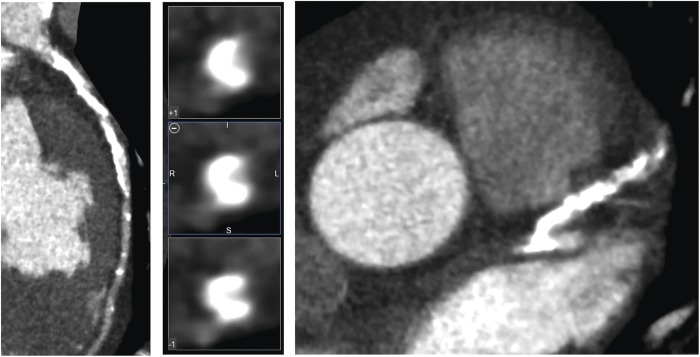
CT coronary angiography images of a heavily calcified left anterior descending artery. The blooming artifact from such heavily calcified plaque can lead to differences in observer classification of stenosis severity.
